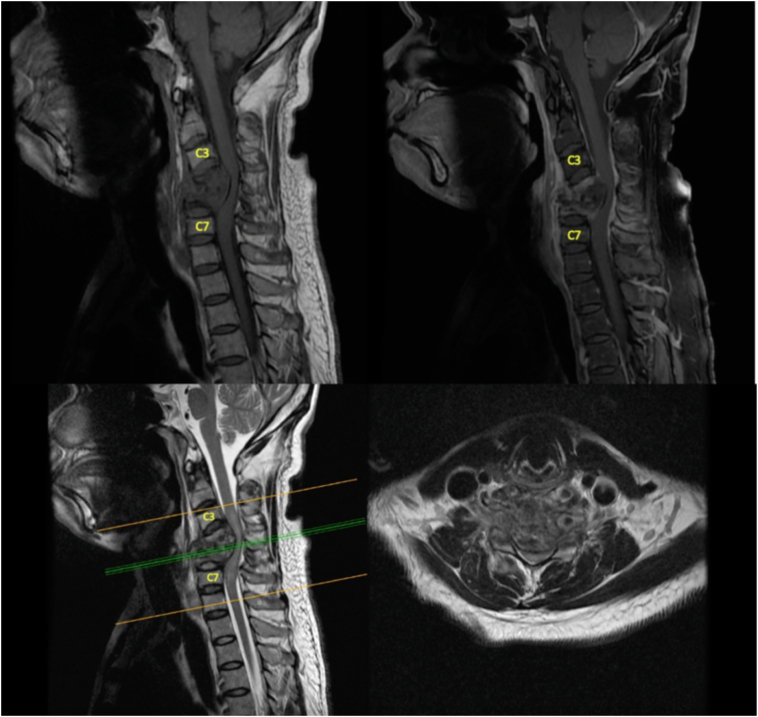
Cervical MRI, T1 with contrast and T2 Sequence.
